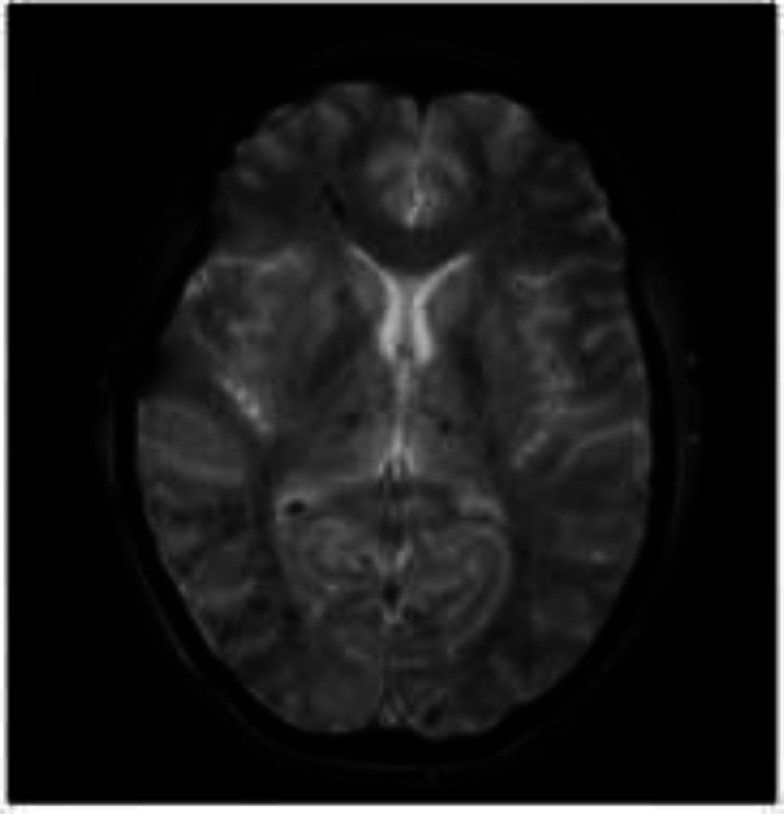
Brain MRI showing the starfield appearance
